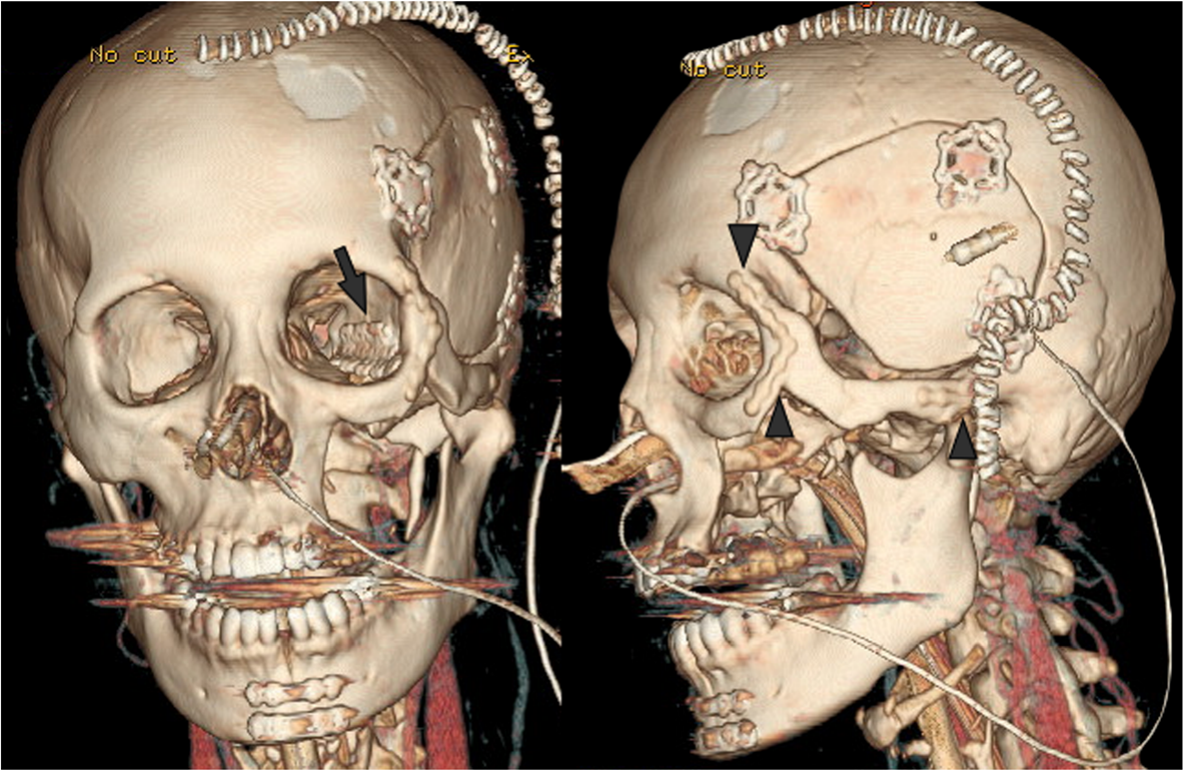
Postoperative computed tomography images. *Arrow* indicates titanium mesh applied on the lateral wall of the orbit. *Arrow heads* indicate the absorbent plate. The orbital rim and zygomatic arch were preserved
